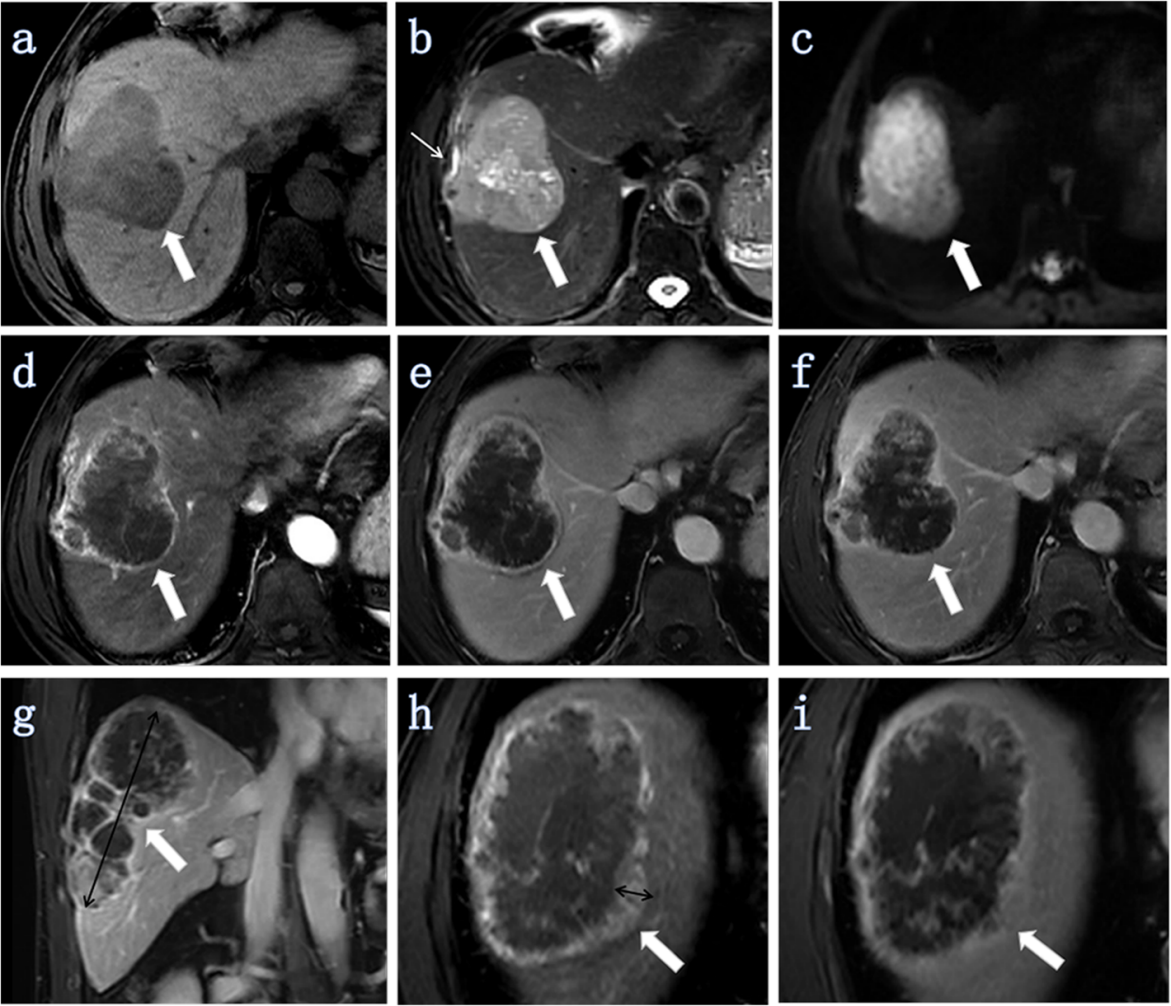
ICC (white thick arrow) with MVI in a 60-year-old man (tumor grade, G3; CA19–9, ≥37 U/ml (155.6 U/ml)). Intrahepatic duct dilatation and visible hepatic artery penetration did not appear in any images of this lesion. (a-f at the same level). a Axial T1W-FS image showing a tumor located in the right lobe of the liver. The tumor morphology was irregular. b Axial T2W-FS image showing the retraction of the hepatic capsule next to the tumor (white thin arrow) and the tumor was unevenly high signal. The signal of the edge was slightly higher while the center signal was very high. c Axial DWI (b = 500 s/mm^2^) showing an uneven higher signal without the target sign. d Axial arterial phase image showing that the tumor exhibited edged enhancement (ring high signal). e Axial portal venous phase image. f Axial delayed phase image showing that the enhancement ratio of the lesion was 0 ≤ R < 1/4. g Coronal portal venous phase image showing that the maximum diameter of the tumor was 12.13 cm (black double arrow). h Axial arterial phase image showing the maximum enhancement edge thickness was 0.34 cm (black double arrow), and the arterial edge enhancement ratio was 2.8% (0.34/12.13). i Axial delayed phase image on the same scanning level as figure h
